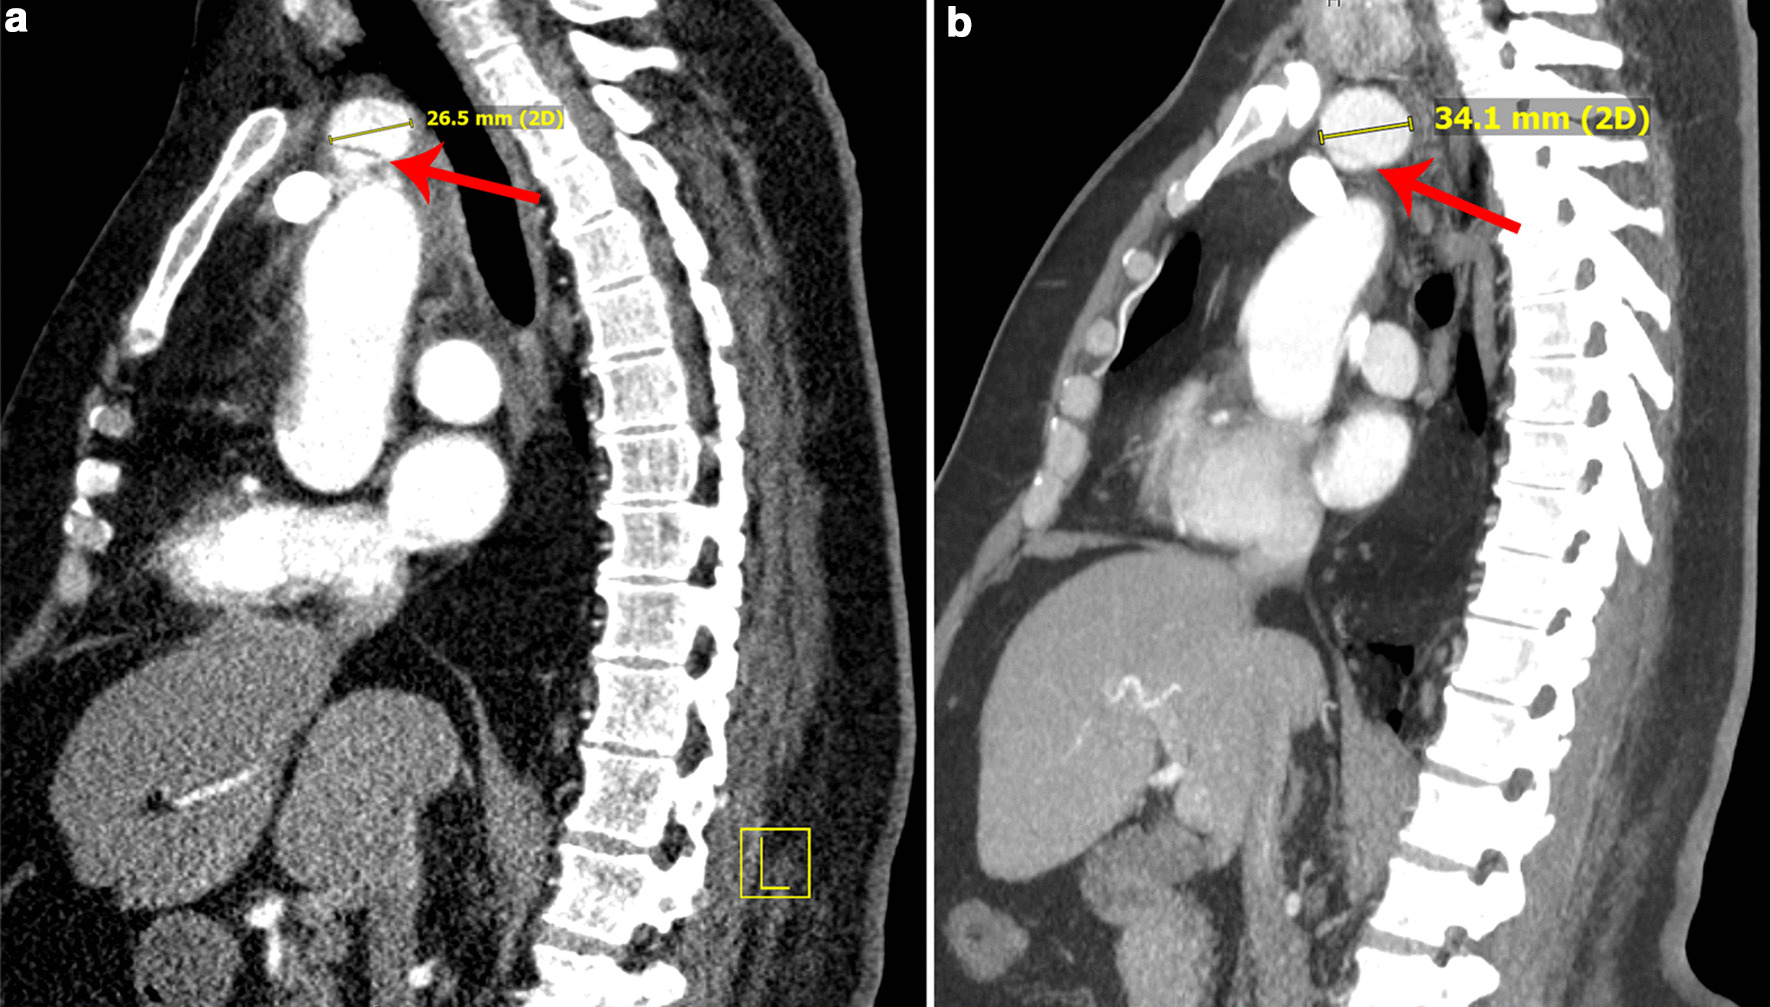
Sagittal computed tomographic angiography of the chest and neck at initial presentation (a) and at 3-month follow-up (b). Imaging reveals slightly increased diameter of the innominate artery after takeoff from the aortic arch compared to diameter at initial presentation. True and false lumens are separated by intimal flap (red arrows, a and b).
